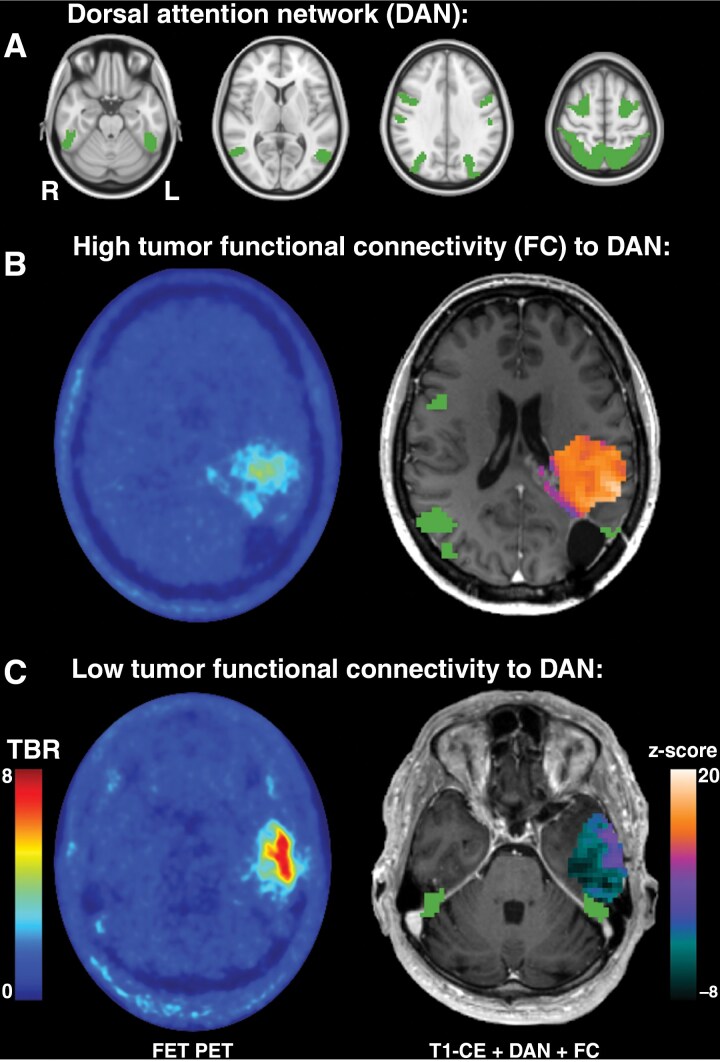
(A) Location of the dorsal attention network (DAN) in the MNI-152 standard space. (B) and (C), exemplary patients with high or low functional connectivity between brain regions infiltrated by metabolic active tumor and the DAN (right) and the corresponding *O*-(2-[^18^F]fluoroethyl)-l-tyrosine (FET) PET images (left). Functional connectivity (FC) of the tumor region was superimposed on the T1-weighted contrast-enhanced (T1-CE) image together with the DAN mask from (A). Abbreviation: TBR, tumor-to-brain-ratio.
